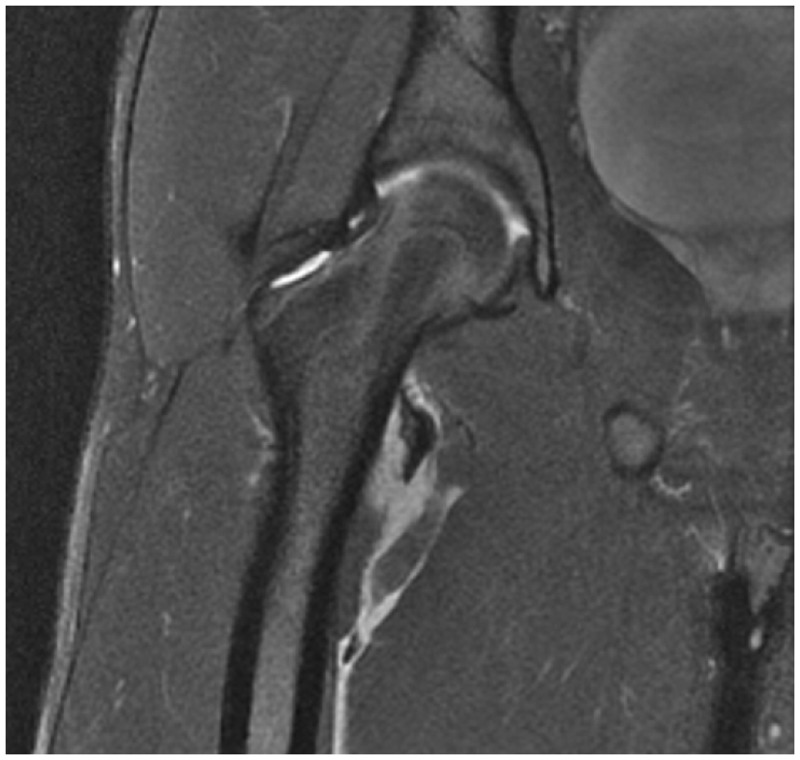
MRI scan showing an avulsion of the right lesser trochanter.
